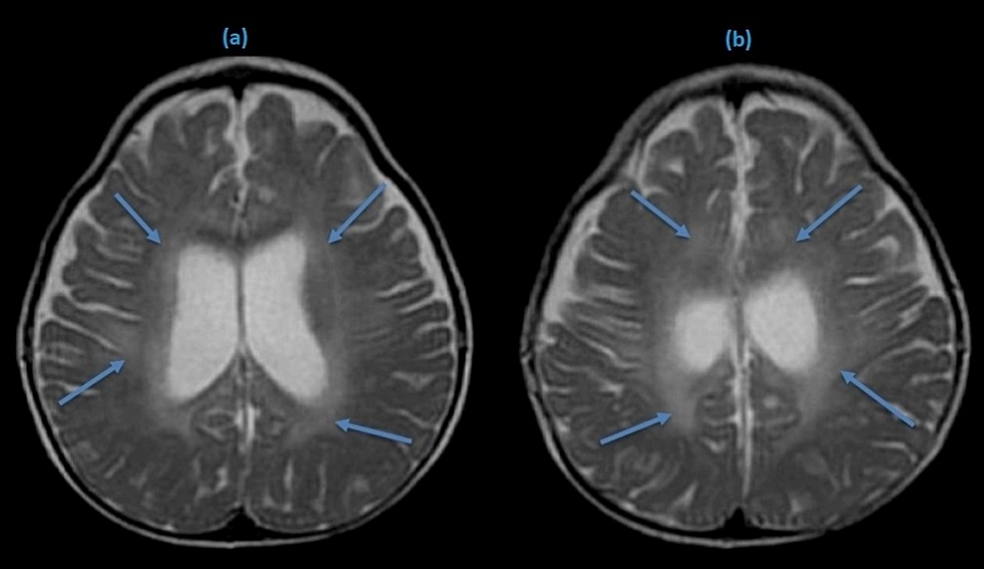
T-2 weighted MRI brain showing hyperintense signals in both hemispheres (blue arrows).
